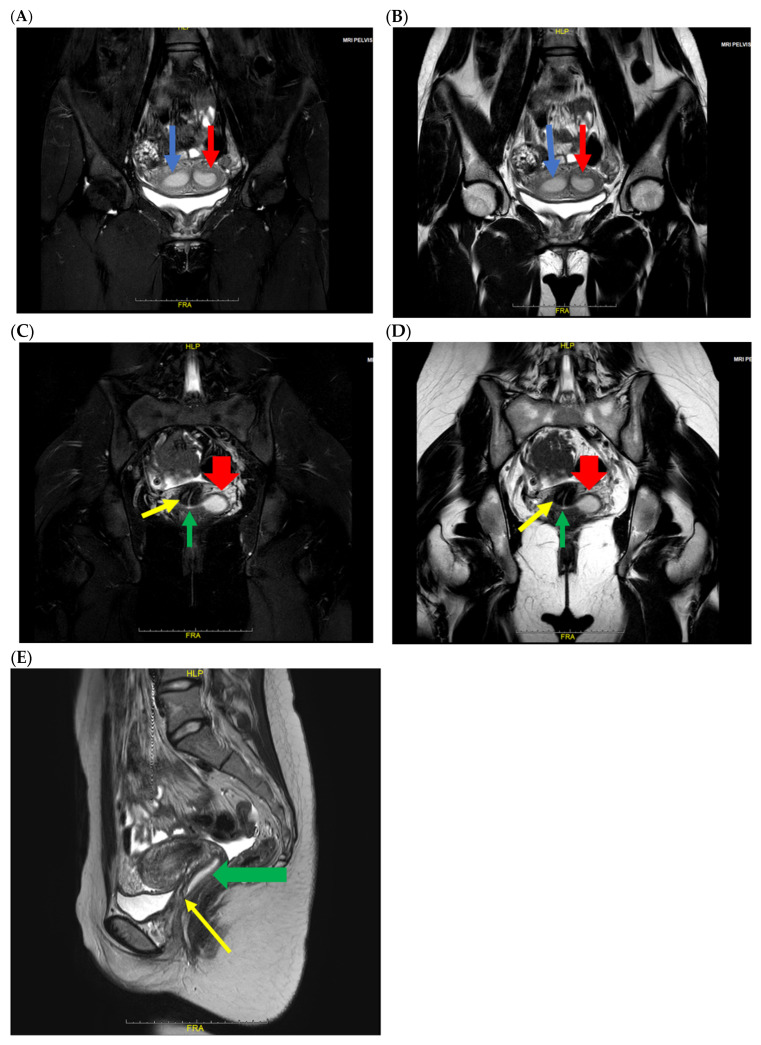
Coronal fat-saturated T2-weighted (A) and coronal T2-weighted (B) MRI images show two uterine cavities (blue arrow: right uterine cavity; red arrow: left uterine cavity). Coronal fat-saturated T2-weighted (C) and coronal T2-weighted (D) MRI images show two uterine cervices. The cavity is filled with hyperintense contents, which are connected to the left side of the uterine cervix (yellow arrow: right uterine cervix; green arrow: left uterine cervix; red arrow: distended hemivagina). Sagittal T2-weighted (E) MRI shows dilatation of the upper vagina and a vaginal septum (yellow arrow: transverse vaginal septum; green arrow: distended hemivagina).
